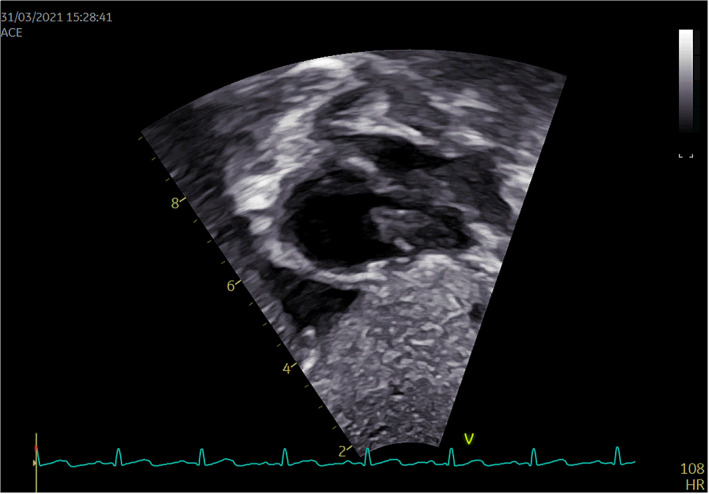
Pretreatment ultrasound 2D dynamic images.
